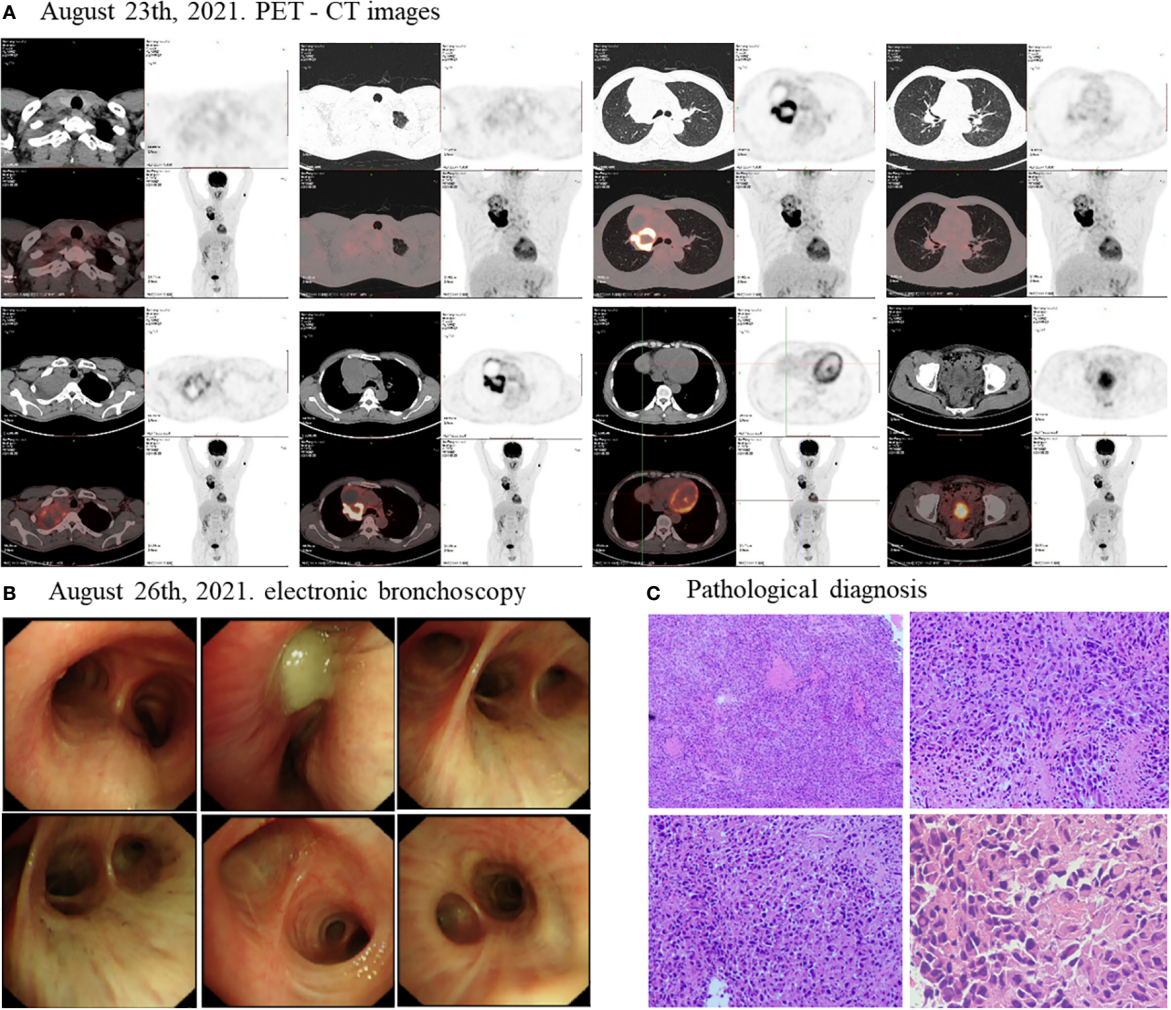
Disease status before treatment. The PET-CT scan (A) showed that before treatment, the size of the lung field from the right hilum to the right upper lobe of the lung large cystic solid mass was 8.6 × 5.9 × 9.9 cm. (B) The electronic bronchoscopy of the patient before treatment. (C) Pathological diagnosis of the patient before treatment.
